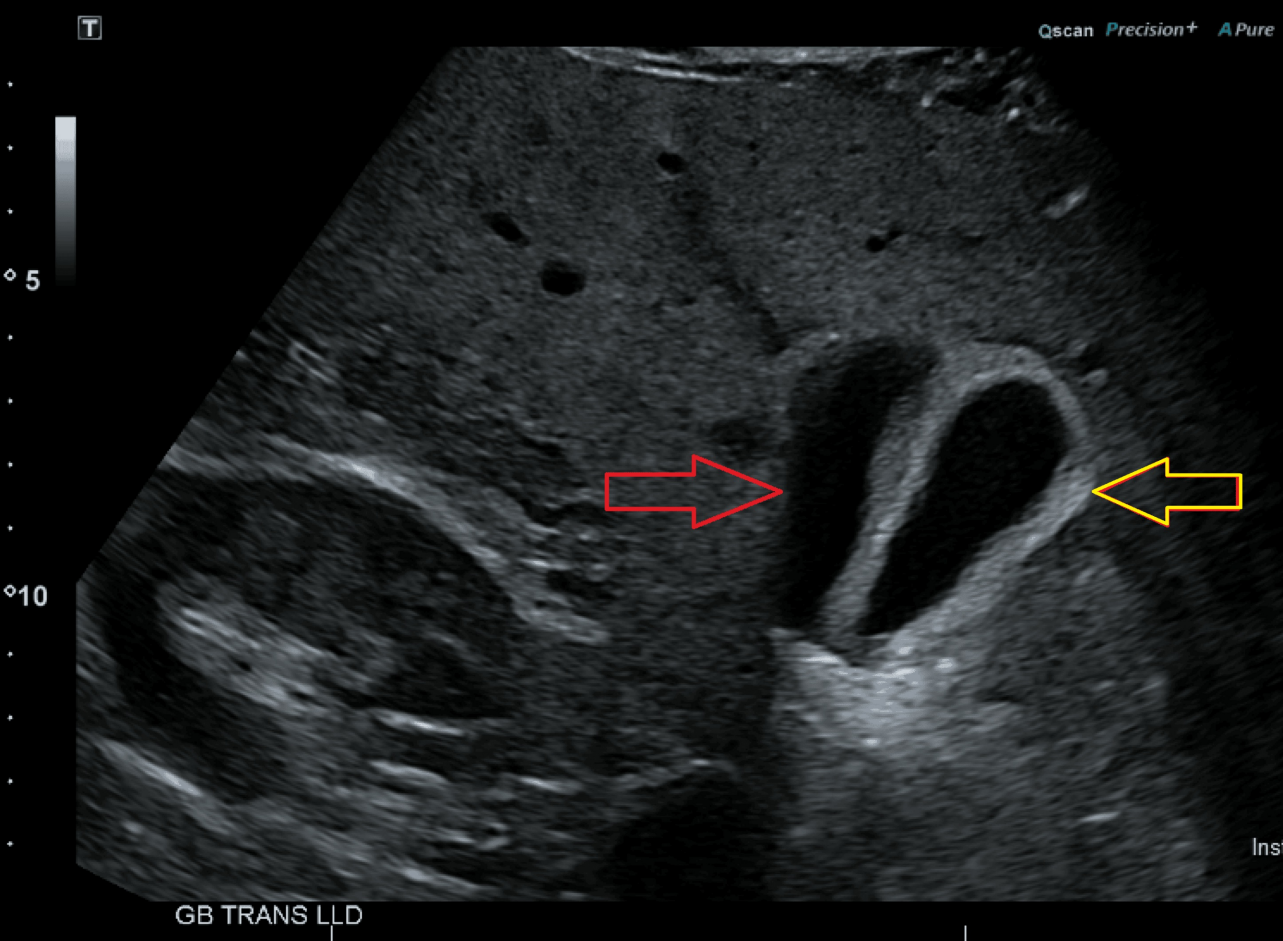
RUQ ultrasound showing duplicated gallbladder. Yellow arrow depicts a thickened gallbladder wall. Red arrow depicts a duplicated gallbladder. RUQ: right upper quadrant.
